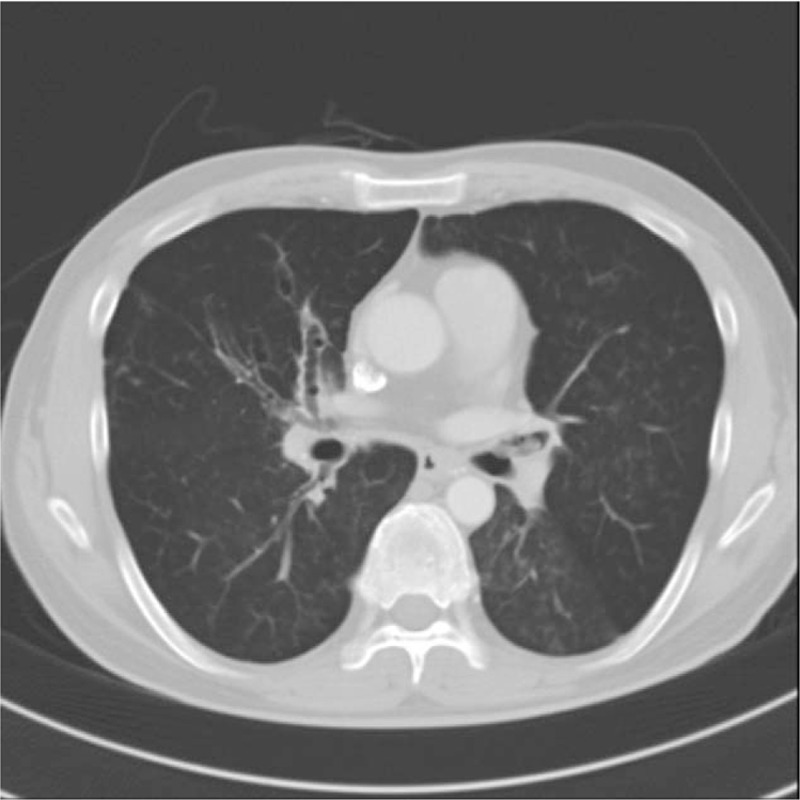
Chest computed tomography performed after the patient coughed up blood showed a suspected blood clot in the left upper lobe bronchus, secretions in both lower bronchi and the left upper lobe bronchus, as well as multiple centrilobular nodules and patchy consolidation in both lungs.
